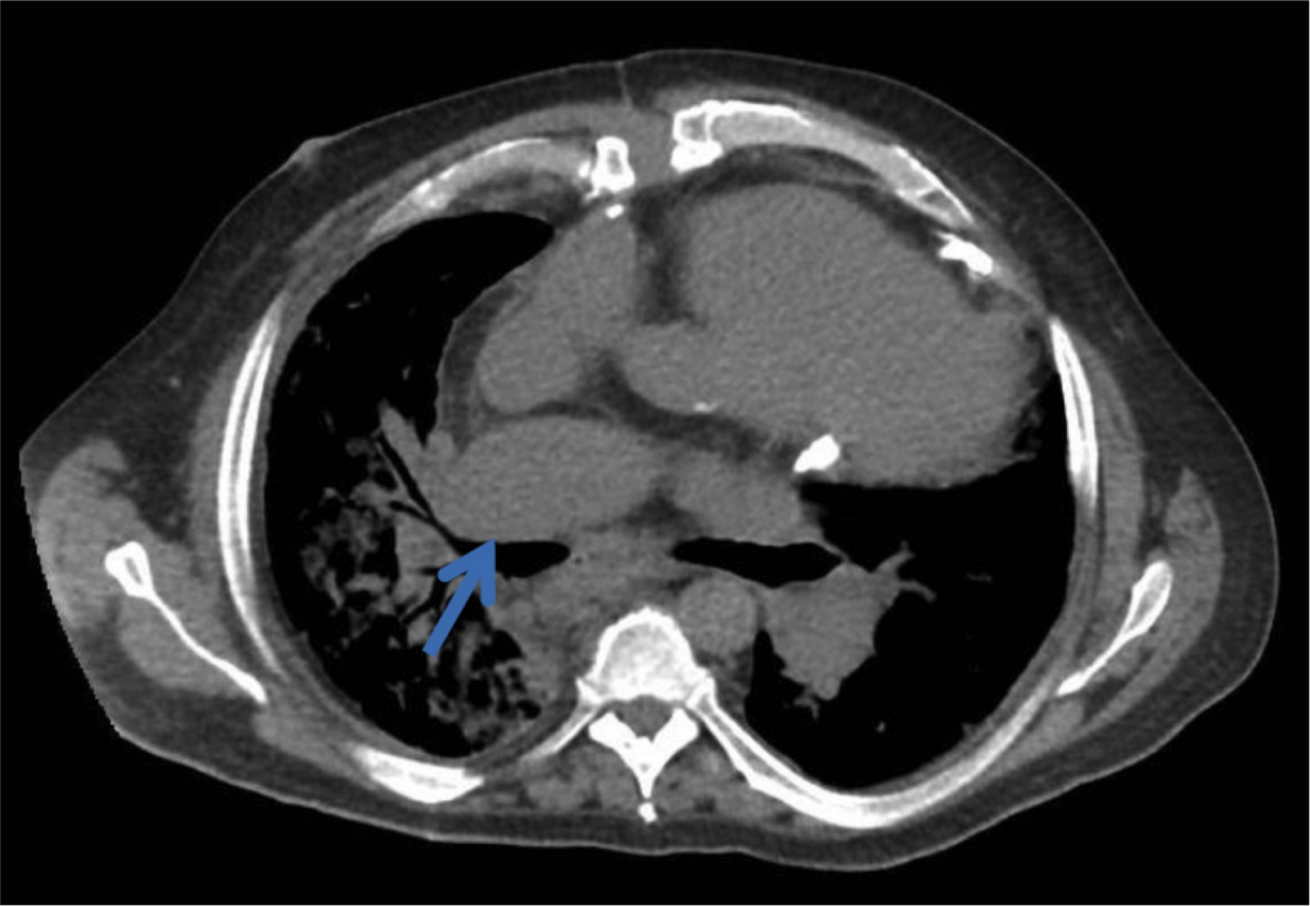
Computed tomography (CT) scan of the chest (abdomen window) showing enlarged right pulmonary artery (PA) causing external compression of right bronchus
